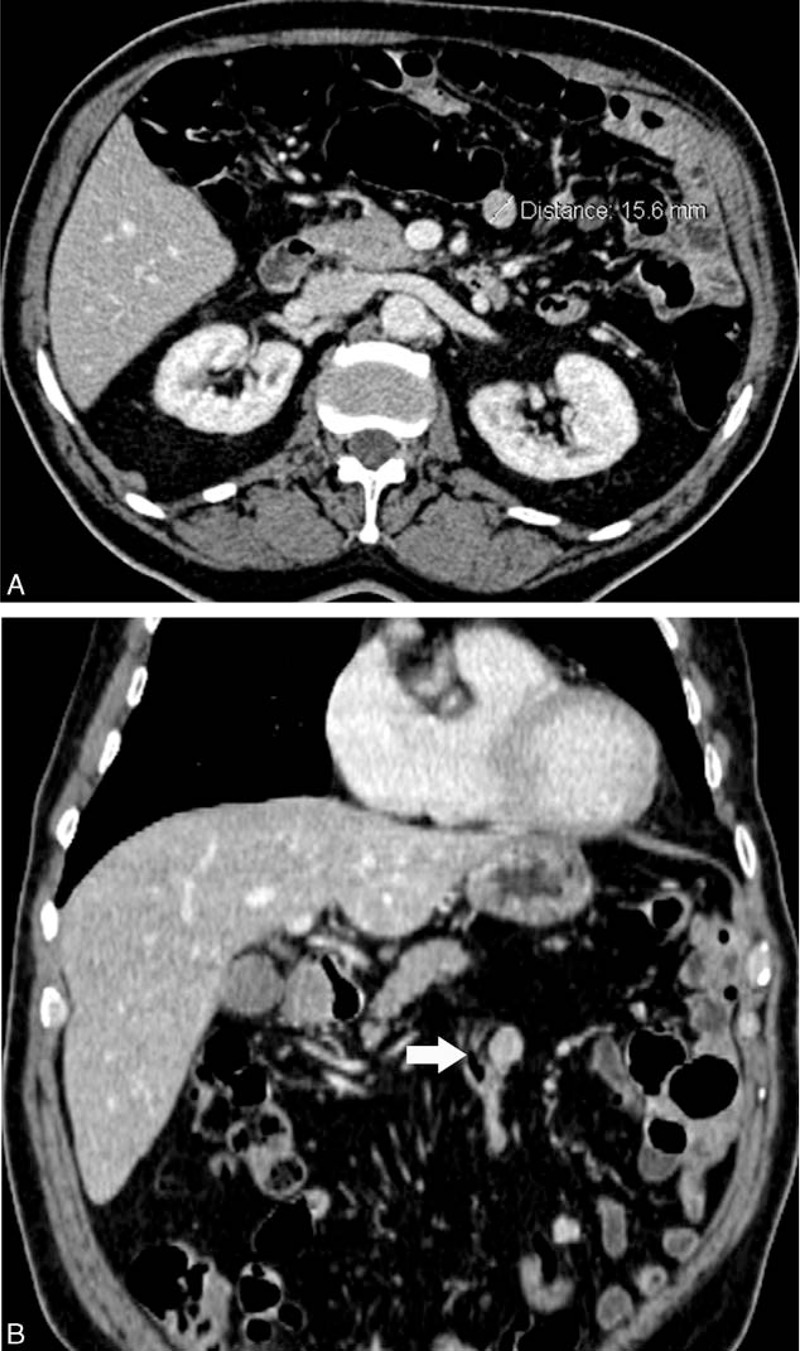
A, Contrast-enhanced CT, axial plane, showing a round mass (approximately 16 mm in its largest diameter) that presents a tight contact surface with a jejunal loop. B, Coronal plane: the white arrow is pointing out the splenosis mass. CT = computed tomography.
